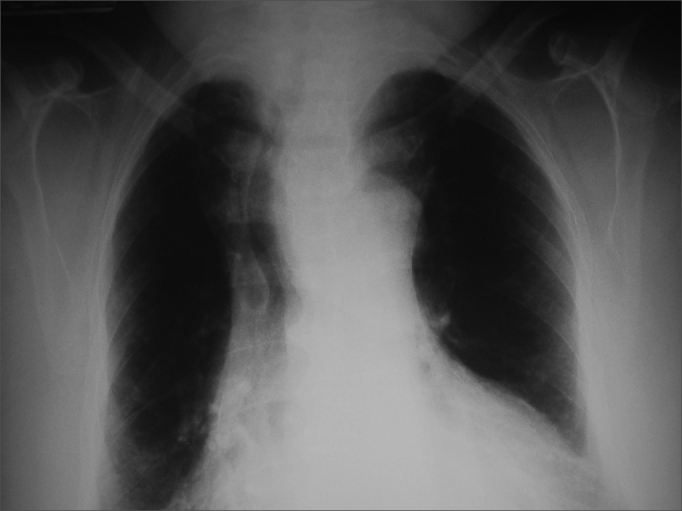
Chest X-ray showing deviated trachea and enlarged upper mediastinum due to substernal goiter.
